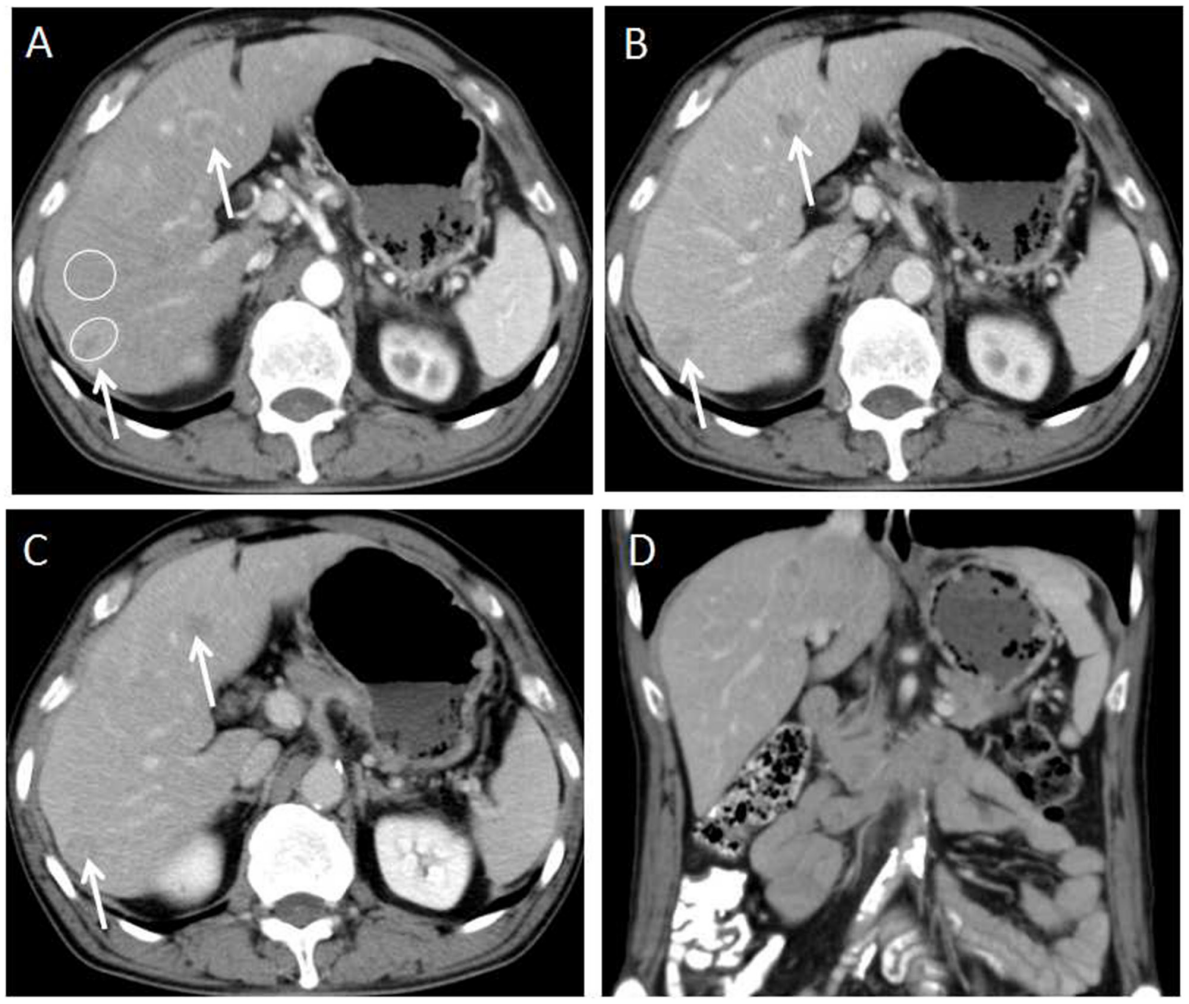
58-year-old male with G2 colon NET (A) Axial CT image on hepatic arterial phase demonstrates multiple hyper enhanced liver metastases in the liver(arrows). Two oval shaped ROIs were placed on the largest lesion and adjacent normal liver. The metastasis-to-liver ratios on hepatic artery phase and portal venous phase were 115.7% and 84.8% respectively. (B, C) Axial CT images on portal venous phase and equilibrium phase show the liver metastases being hypo enhanced that meets the washout enhancement pattern(arrows). (D) Coronal CT image on the portal venous phase shows absent of lymphadenopathy.
